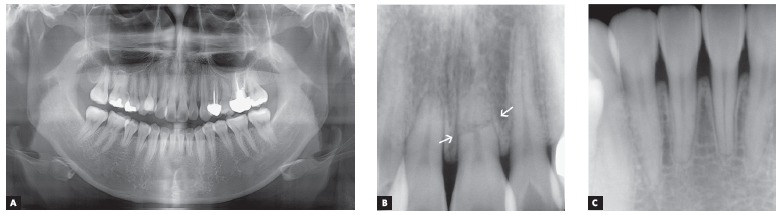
A) Pretreatment panoramic radiograph. B, C) Upper and lower incisors periapical radiographs. White arrows indicate the apical root fracture of the upper left central incisor.
